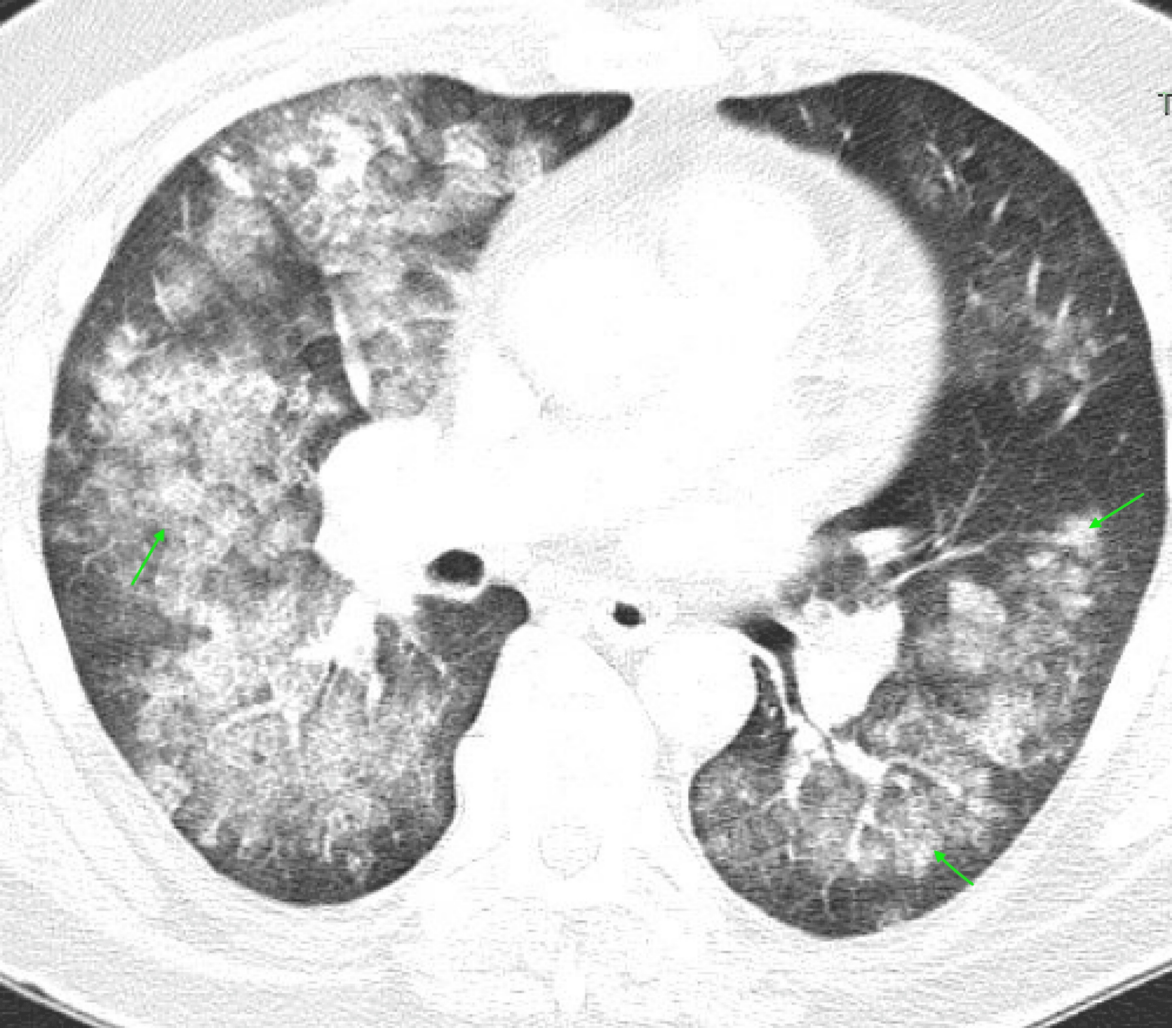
A computed tomography (CT) angiography of the chest Diffuse bilateral perihilar infiltrates with patchy ground-glass opacities (arrows), consistent with pulmonary hemorrhage.
